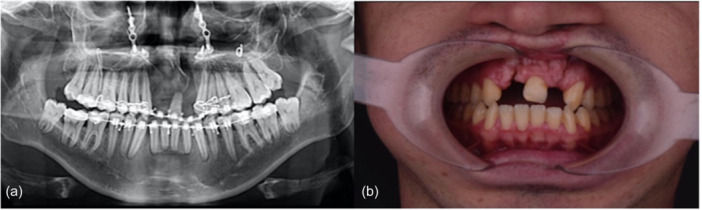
(a) Radiographical and (b) clinical baseline images showing previous surgical repair of bilateral cleft lip and palate with oronasal fistula.
